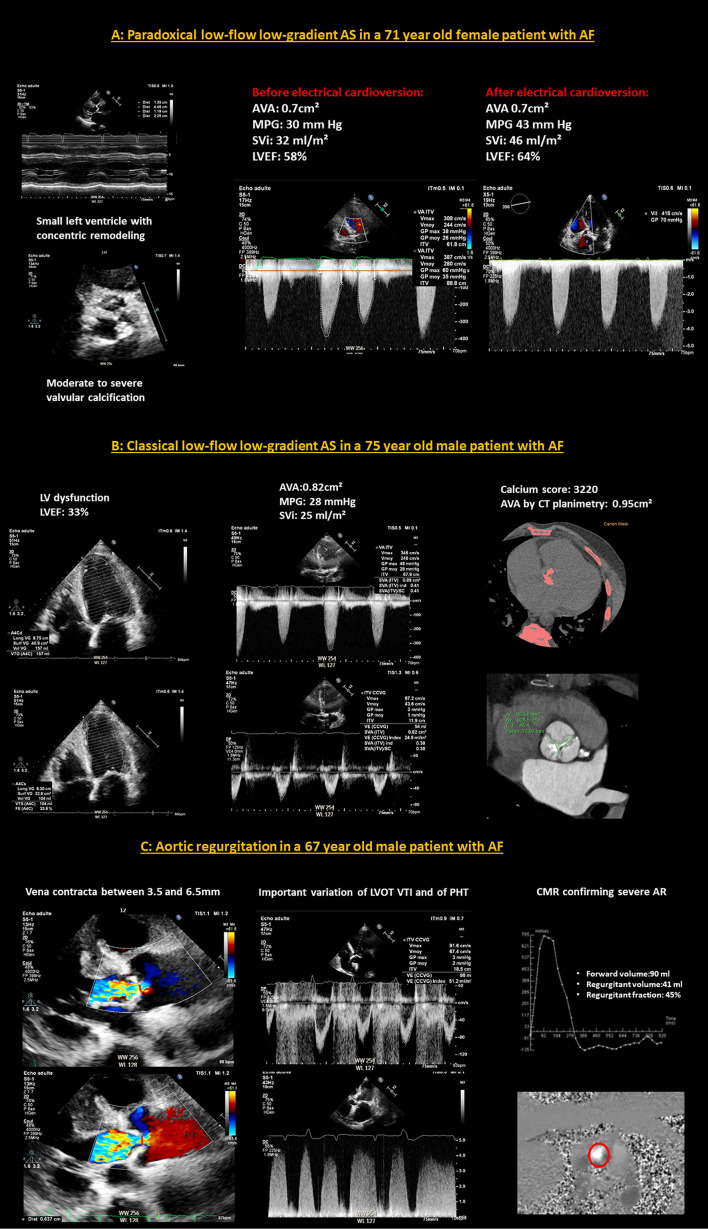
Examples of difficult cases of echocardiographic evaluation of aortic valve disease in patients with AF. (A) A case of paradoxical low-flow low-gradient AS in a 71-year-old female patient with AF. The left ventricle is small with concentric remodeling and preserved LVEF and the aortic valve presents moderate to severe calcifications. There is a clear variation of the aortic flow according to the cardiac cycles. The MPG is low, calculated at 30 mm Hg and the SVi is averaged at 32 ml/m^2^, in favor of a paradoxical low-flow, low-gradient AS. After electrical cardioversion, and the restoration of SR, the cycles no longer fluctuate, the SVi has normalized to 46 ml/m^2^ and the MPG is high at 43 mmHg. The AVA remained stable at around 0.7cm^2^ before and after cardioversion. (B) A case of classical low-flow low-gradient AS in a 75-year-old male patient with AF. There is a left ventricular dysfunction, with a LVEF estimated at 33%. The flows vary slightly according to the cardiac cycles. The MPG is low, averaged at 28 mmHg as well as the SVi which is estimated at 25 mml/m^2^. The cardiac CT-scan is in favor of a severe AS because the calcium score is very high at 3,220 and the aortic valve planimetry finds 0.95cm^2^. (C) A case of aortic regurgitation in a 67-year-old male patient with AF. It is difficult to distinguish between a moderate and a severe AR on echocardiography because of the marked variation of the parameters (vena contracta, LVOT VTI and PHT) between cardiac cycles. Evaluation by phase-contrast CMR, which is less disturbed by AF than echocardiography, leads to the conclusion of severe AR with a calculated regurgitation fraction of 45%. AF, atrial fibrillation; AR, aortic regurgitation; AS, aortic stenosis; AVA, aortic valve area; CMR, cardiac magnetic resonance; LVEF, left ventricular ejection fraction; LVOT VTI, left ventricular outflow tract velocity time integral; MPG, mean pressure gradient; PHT, pressure half time; SR, sinus rhythm; SVi, stroke volume index.
